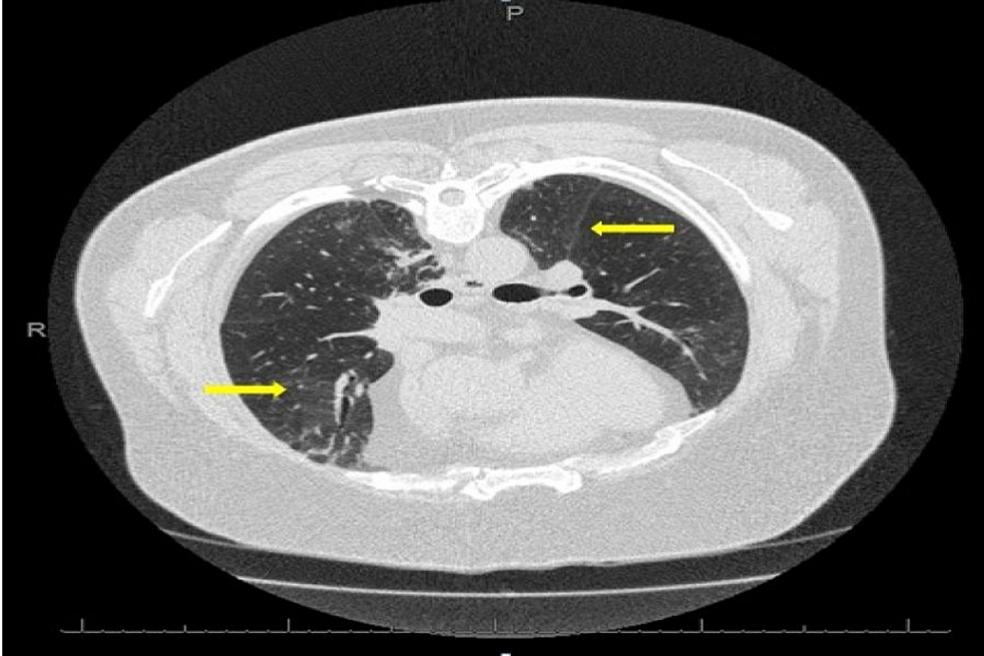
HRCT-axial view There is diffuse and patchy ground-glass attenuation (yellow arrows) with tiny nodules in the upper and mid zones, and there are multifocal areas of peripheral consolidation with tractional bronchiolar dilatation within both lower lobes. HRCT: high-resolution computed tomogram
